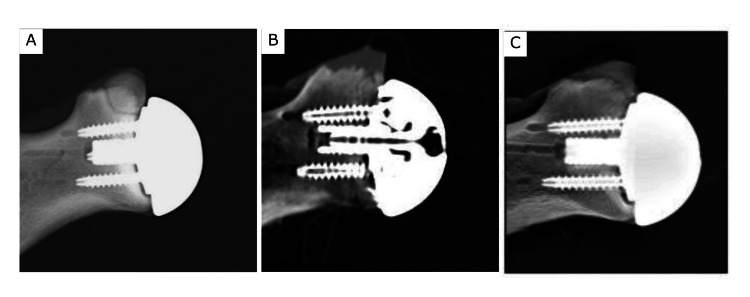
Control model a. radiographic evaluation, b. coronal CT image, and c. tomosynthesis.
